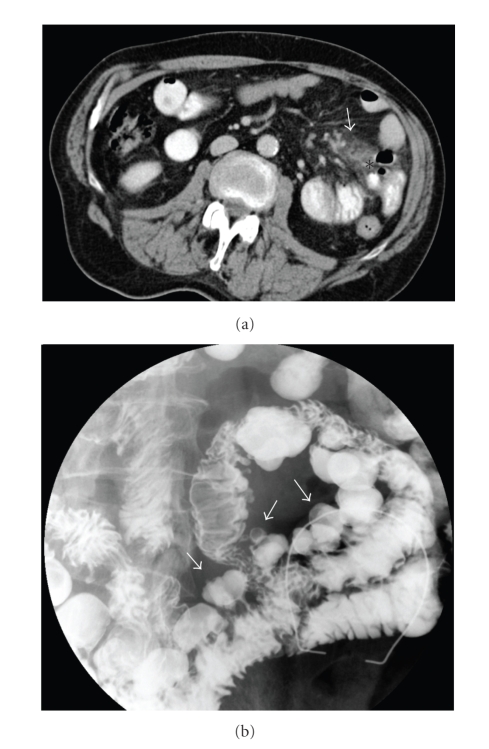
(a) Transverse CT section through the upper abdomen reveals localised inflammatory changes in the left upper quadrant (arrow) accompaigned by extraluminal air bubbles at the mesenteric side of the jejunum (asterisk). (b) Small-bowel barium follow-through exam performed three weeks after the acute onset confirms the presence of multiple jejunal diverticula (arrows).
